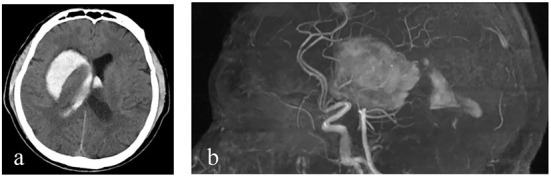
Imaging findings. (a, b) Cranial CT and cranial MRA show an intracerebral hemorrhage in the right basal ganglia and in bilateral lateral ventricles.
